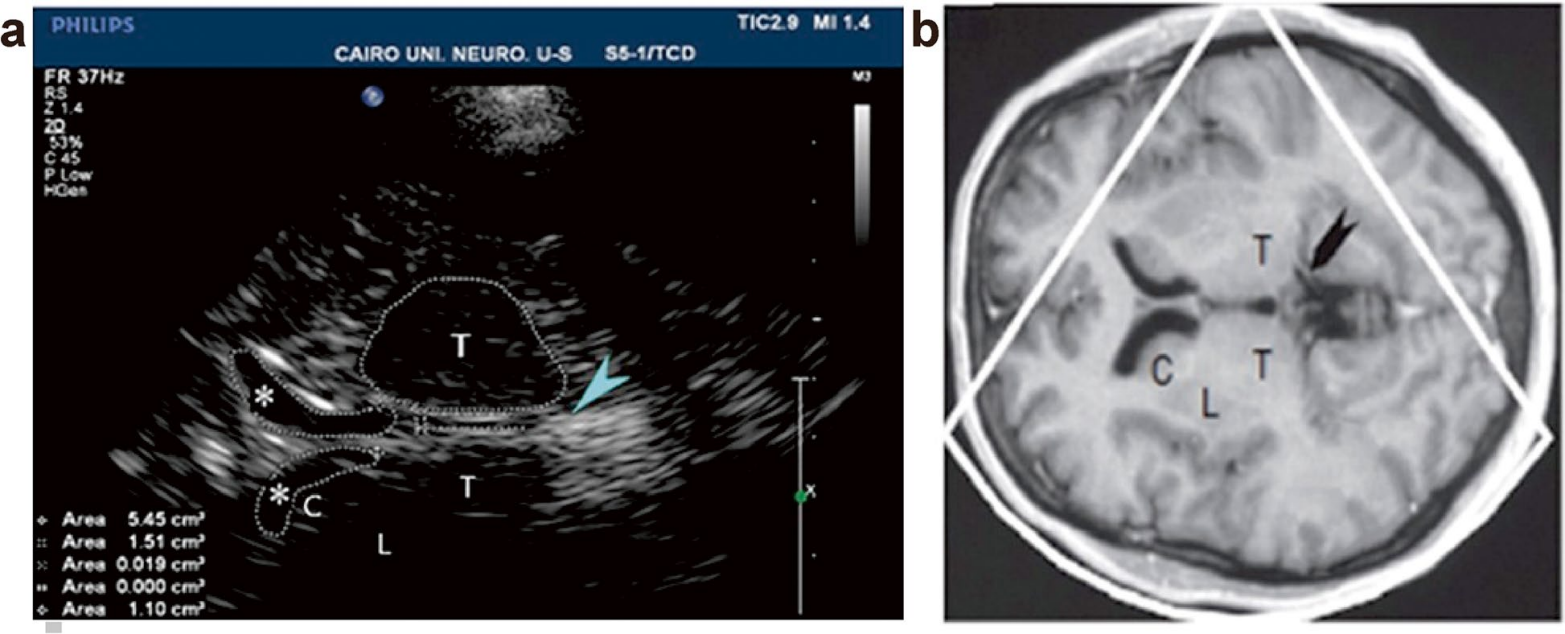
TCS axial view at the thalamus level (a) and corresponding MRI picture at the same level (b); T = thalamus; * = frontal horn of lateral ventricle; C = caudate nucleus and lentiform nucleus; arrowhead = pineal gland (hyperechogenic landmark)
